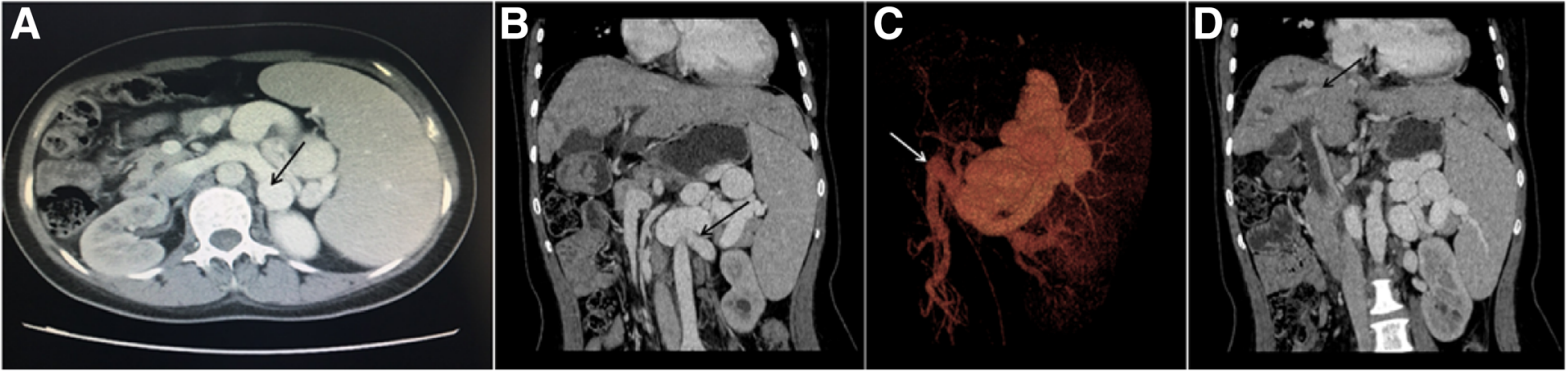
Axial, coronal and three-dimensional CT images show type II Abernethy malformation in the patient. (a and b) A side-to-side shunt between the splenic vein and the left renal vein is visible in axial and coronal CT images (black arrow). c 3D vessel reconstruction shows a normal main portal vein formed from the splenic and superior mesenteric veins (arrow). d The portal vein appears slender in the coronal CT image (arrow)
